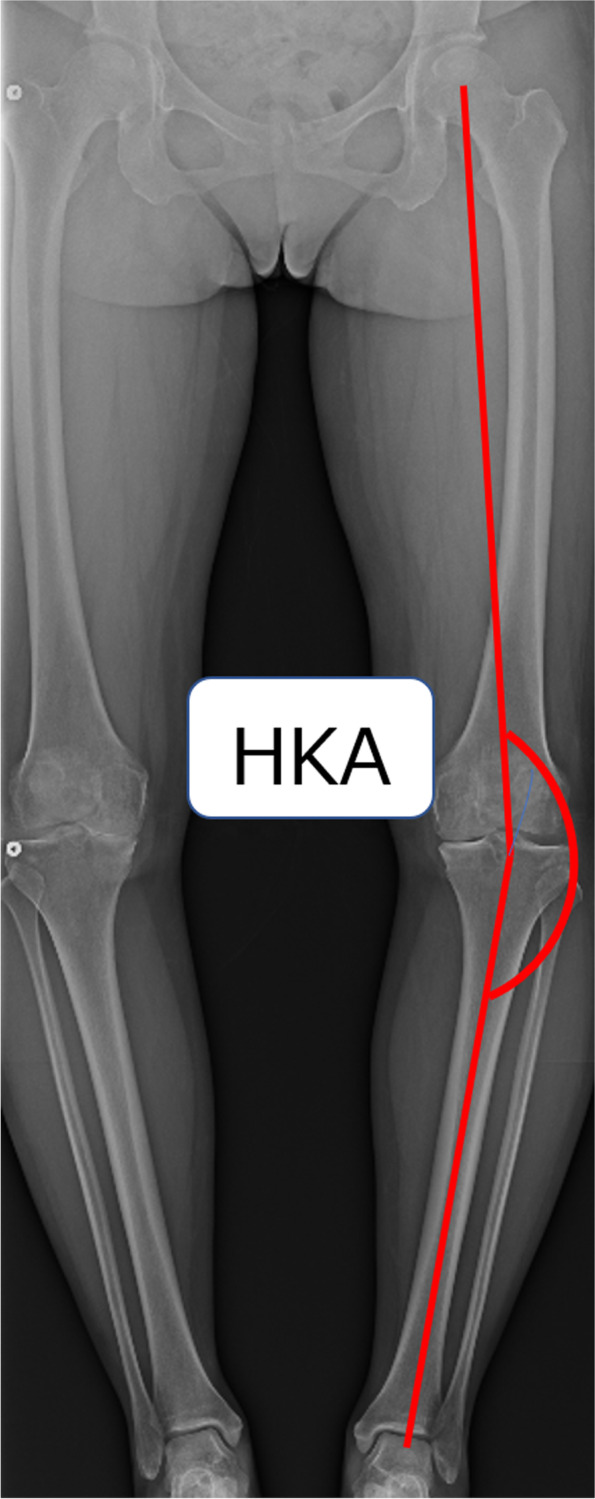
Measurement of the HKA on whole-leg standing radiographs. HKA, hip-knee-ankle angle
